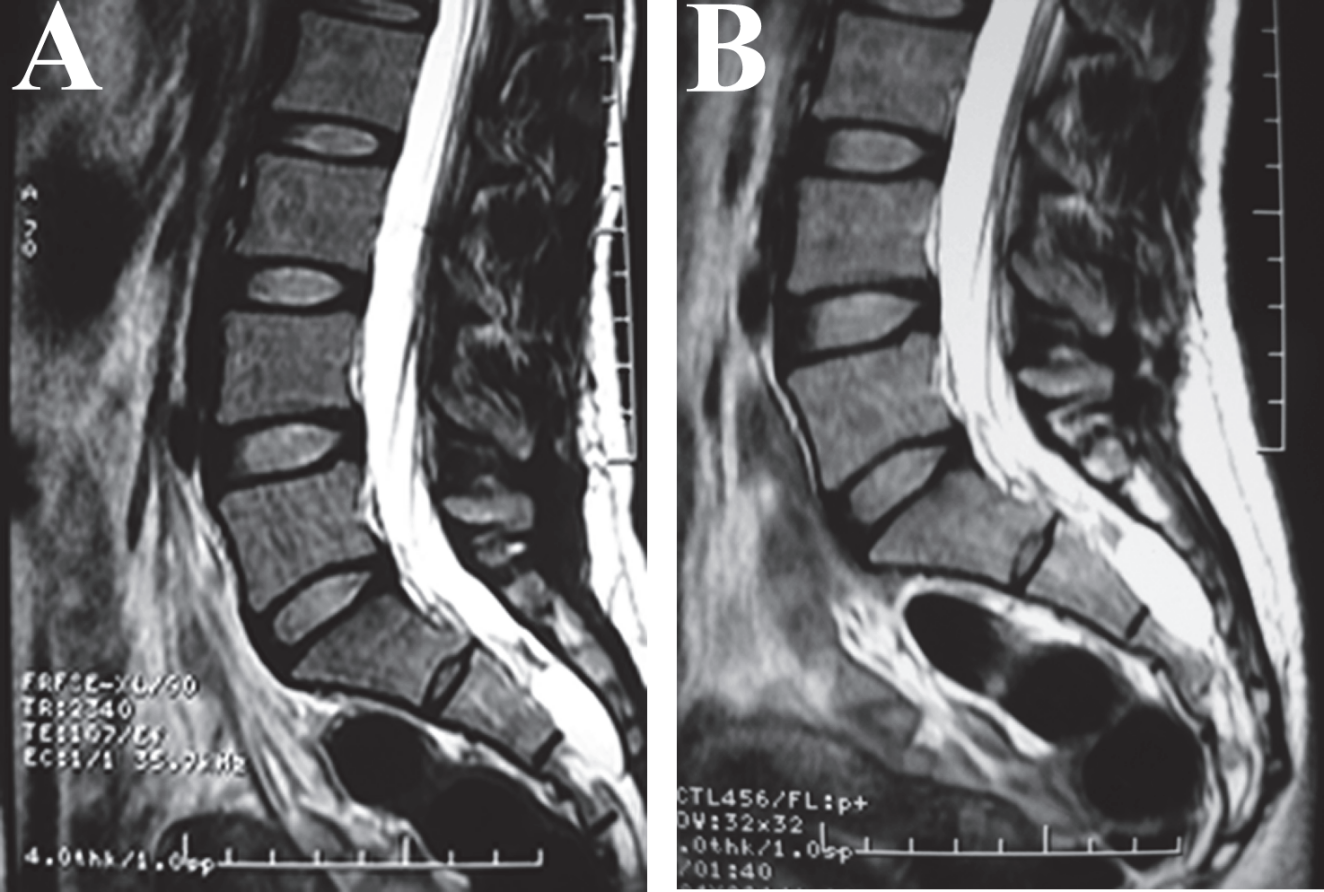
MRI study of no change of the cyst. A. Sagittal T2WI MRI shows a single cyst located in S2-S3 before fibrin gel therapy. B. Sagittal T2WI MRI obtained 10 months after operation shows that the size of the cyst did not change.
